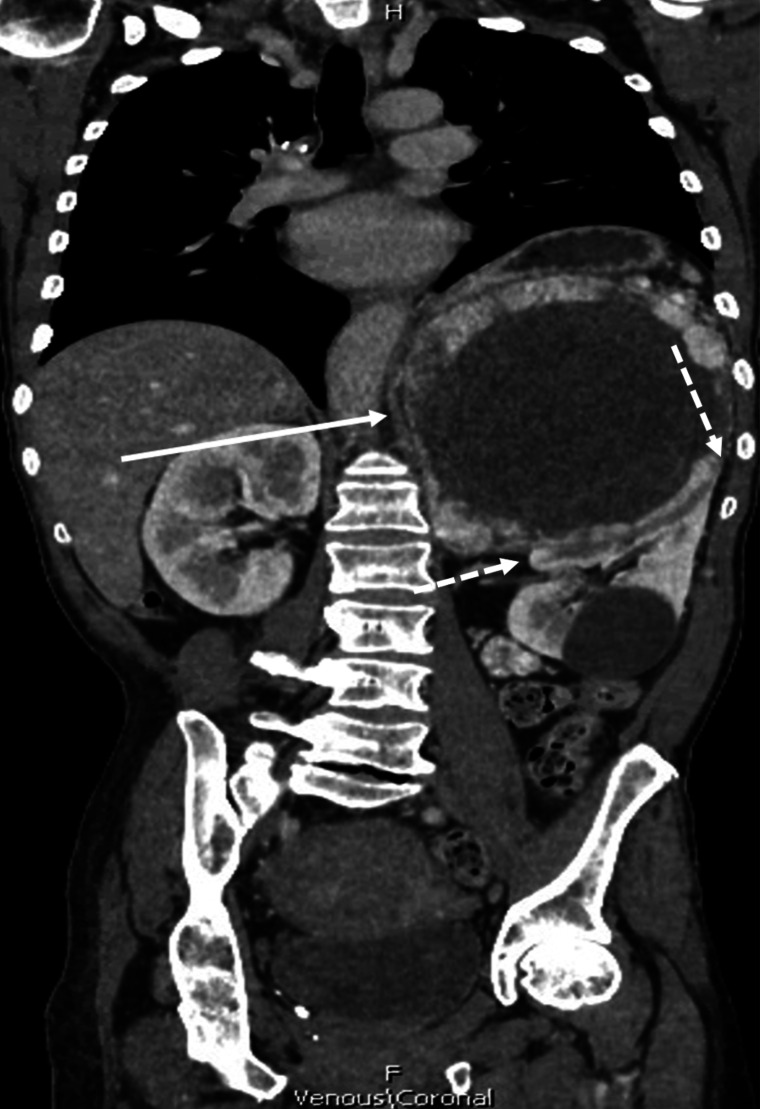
Abdominal computed tomography scan (coronal image) showed a centrally necrotic giant pheochromocytoma (solid arrow) overlying the left kidney, measuring 15.9 × 12.7 × 14.7 cm. “Claw sign” can be seen as the tissue circumscribing the bottom of the giant pheochromocytomas, indicated by dashed arrows.
